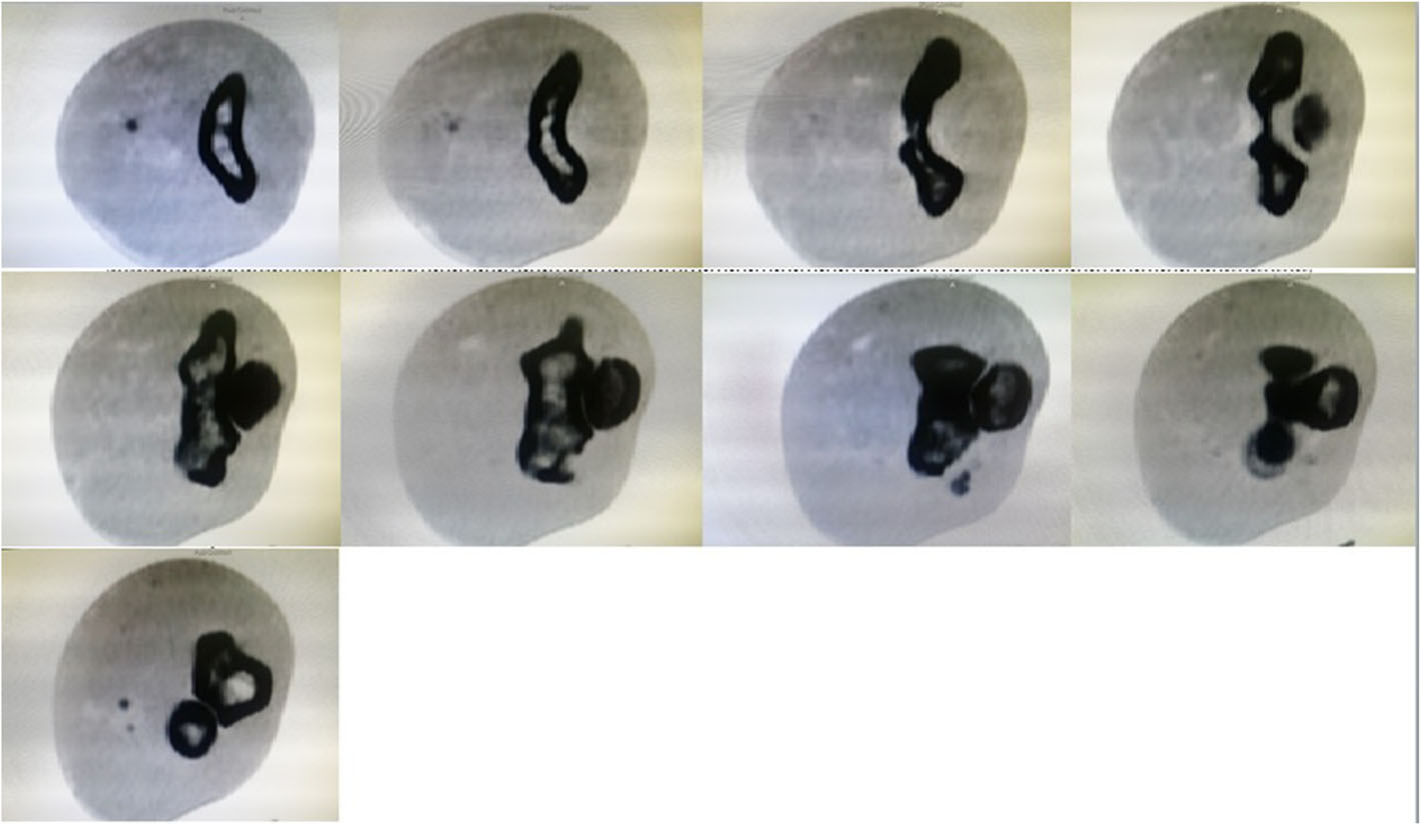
Axial view computed tomography of left elbow
